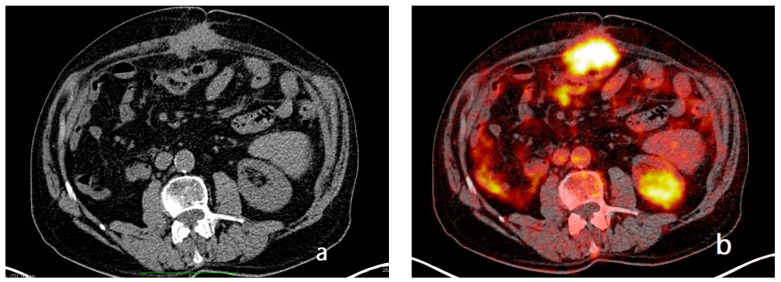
Axial non-contrast-enhanced computed tomography through the mid-abdomen demonstrates a nodule of peritoneal carcinosis on the middle abdominal wall (a) in a 68-year-old man. 18F-fluorodeoxyglucose PET with low-dose attenuation-correction-computed-tomography-fused image demonstrates its fluorodeoxyglucose uptake (b).
